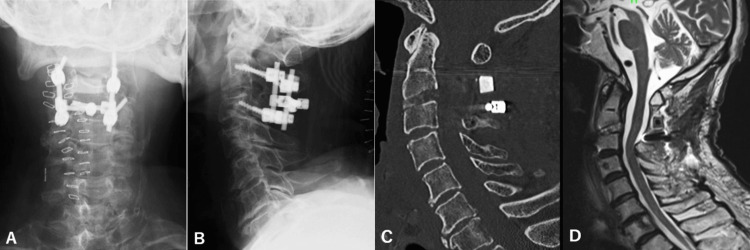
Plain anterior-posterior (A) and lateral (B) X-rays and CT (C) on the same patient after completion of anterior and posterior fusion, showing realignment of his cervical spine, the kyphotic angle of 3 degrees. MRI (D) revealing complete decompression of the spinal cord.
